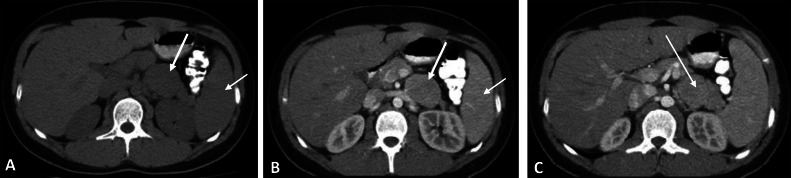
Abdominal CT scan without contrast (A) revealed a homogenous density retroperitoneal mass(arrows) adjacent to the pancreatic tail. Contrast-enhanced imaging (B, C) showed a similar enhancement pattern to the spleen, suggesting an accessory spleen as a potential differential diagnosis.
